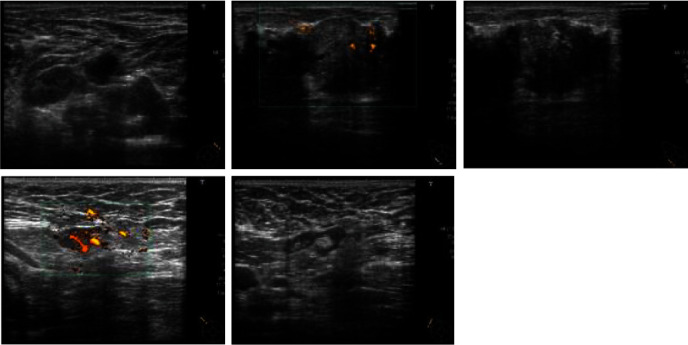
Ultrasonography revealed an irregular hypoechoic lesion at the lower outer aspect of the right breast, with a maximum diameter of ~39 mm, and bilateral lymphadenopathy.
